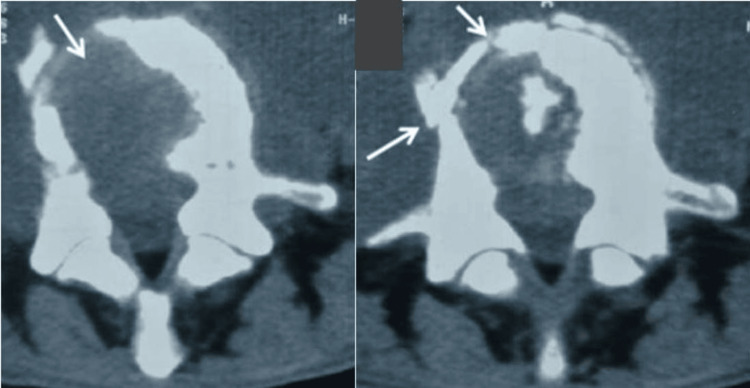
Lumbar spine CT scans: osteolysis of the vertebral body of L5 with bone fragmentation and anterior and posterior cortical rupture
